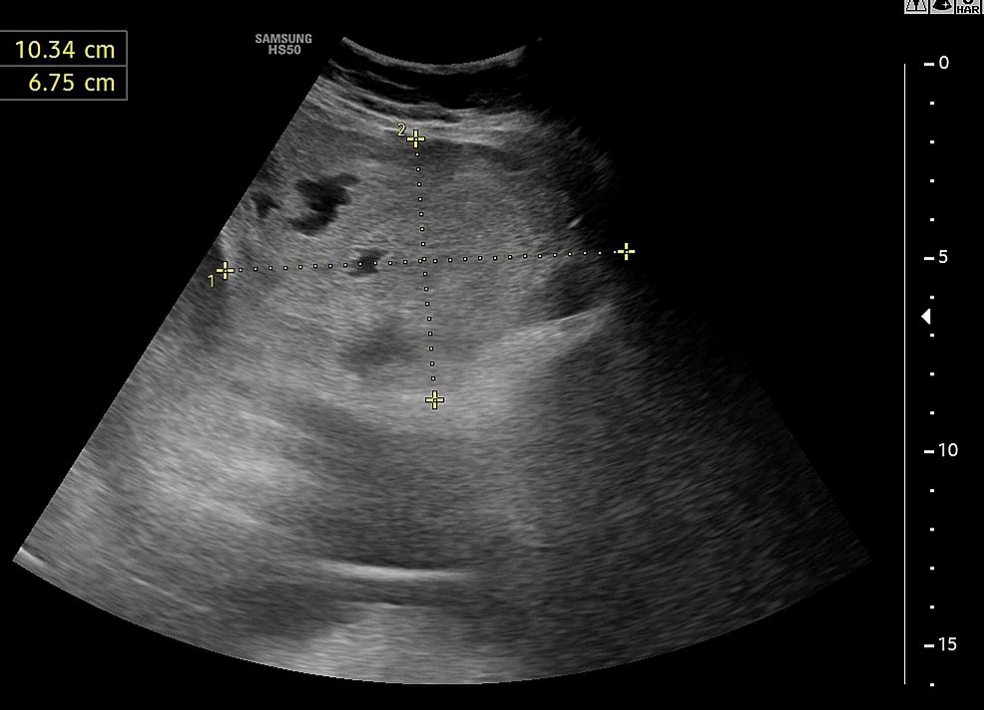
POCUS examination of the paracentesis site. POCUS, point-of-care ultrasonography
